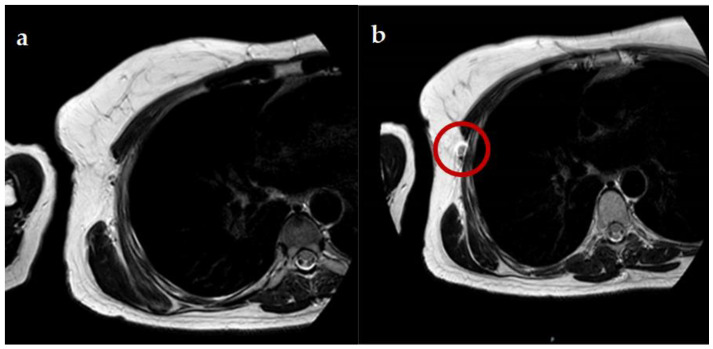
(a,b). Visualization of SLN with MRI before and after SPIO. In an enhancement of the SLN is visualized after injection of SPIO. The red circle visualizes the enhanced SLN after the injection of SPIO.
